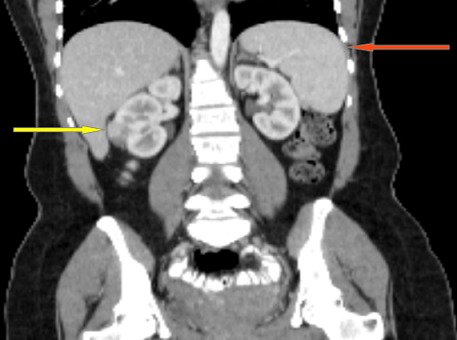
Pre-surgery coronal abdomen CT: shows spleen (red arrow), right renal mass (yellow arrow)
